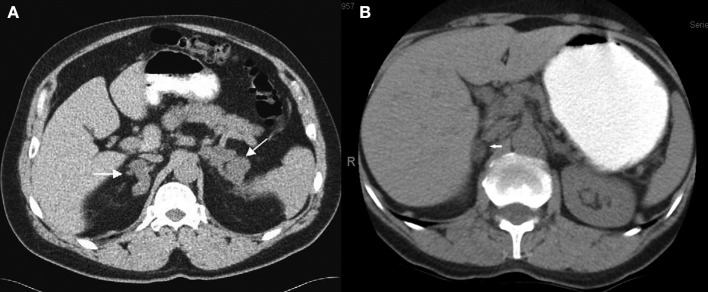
Abdominal CT scan with contrast showing the heterogenous presentation of the disease. (A) Bilateral adrenal nodules case #14. (B) Unilateral adrenal nodule—right gland case #20.
